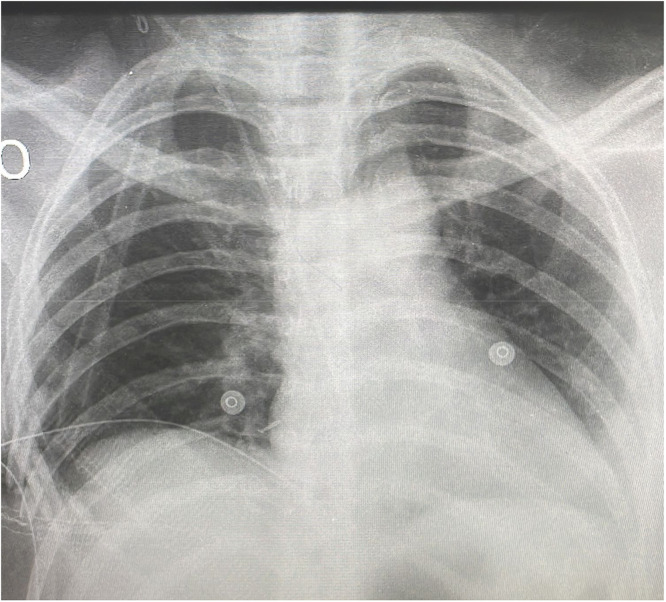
Chest X-Ray showing prosthesis radiolucency.
